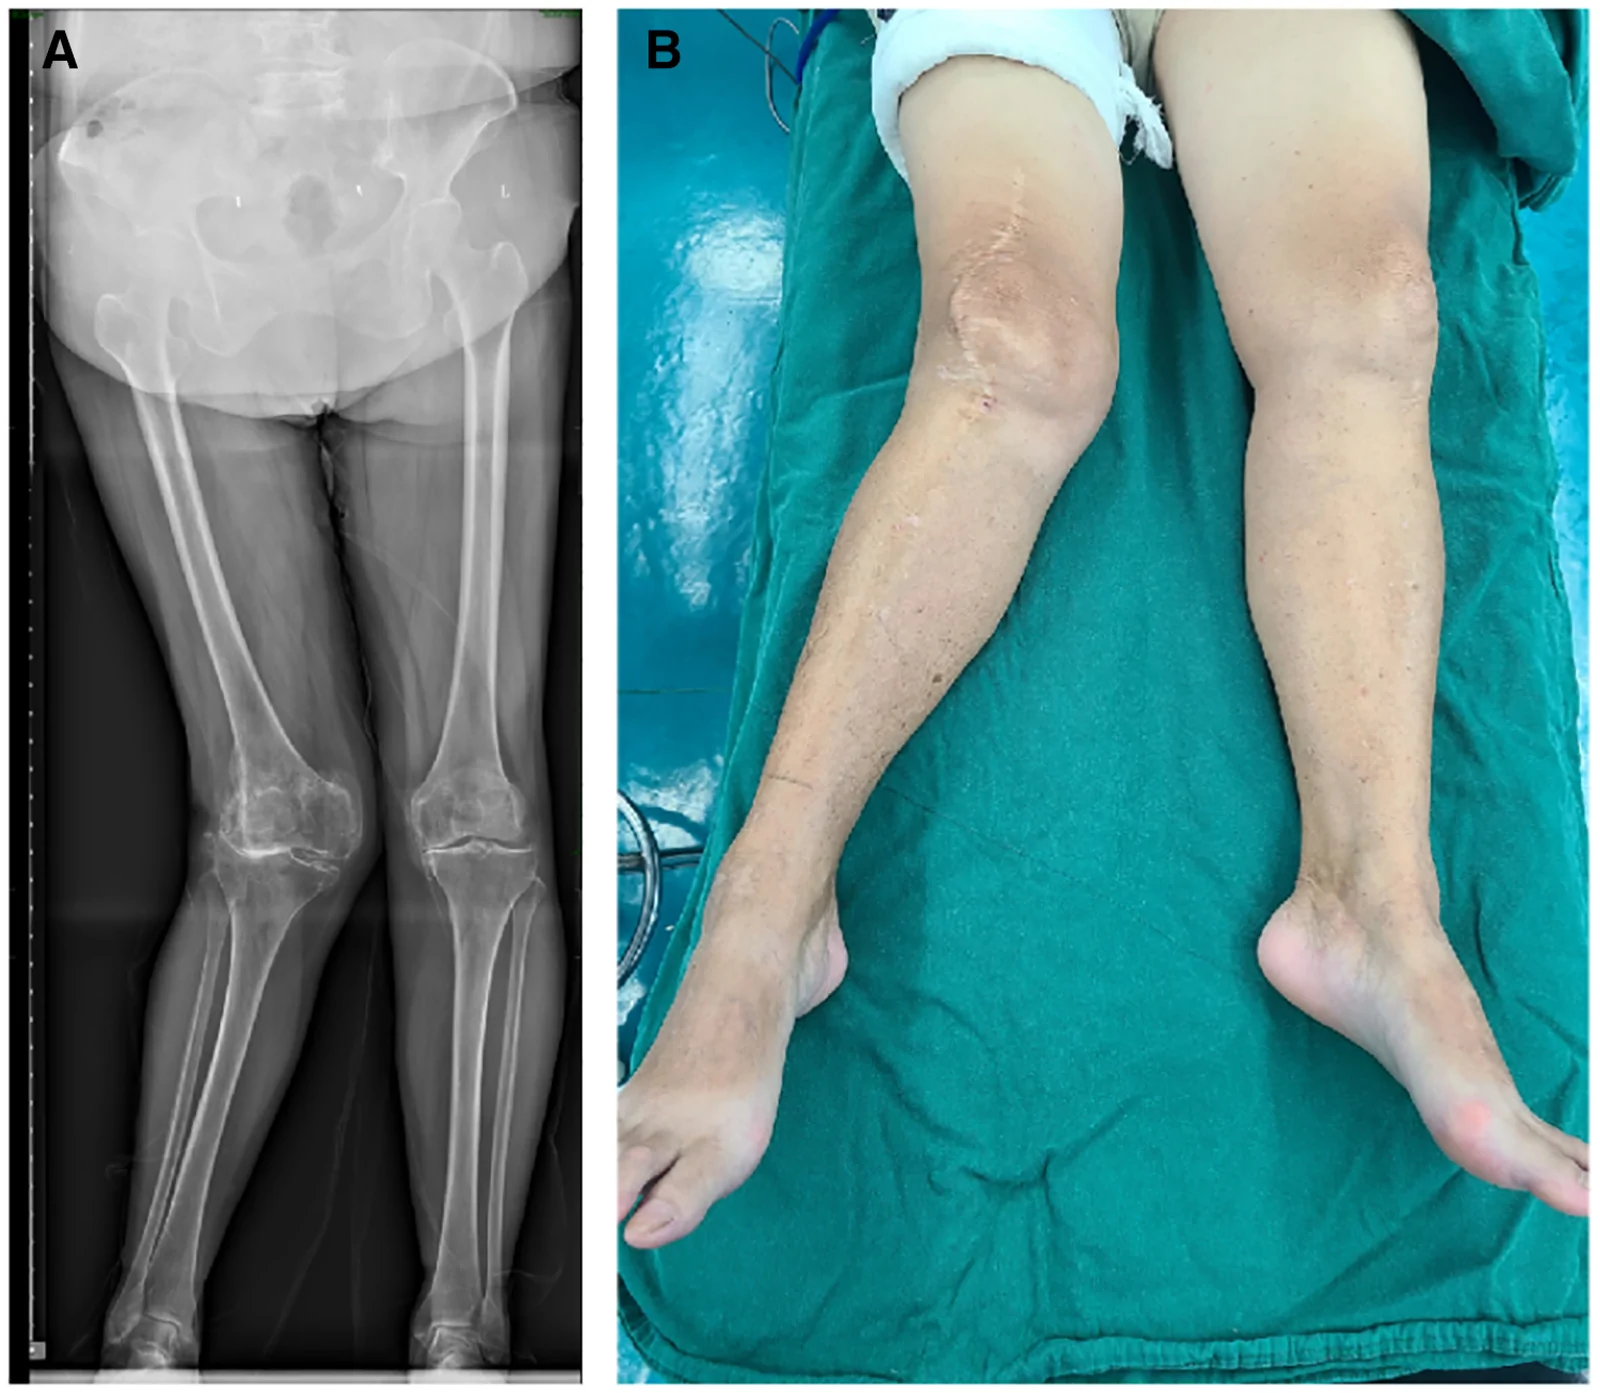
(A) Preoperative full-length radiograph of both lower extremities shows lateral femoral condyle defect and valgus deformity. (B) Preoperative physical examination revealed a surgical scar on the right lateral knee.
